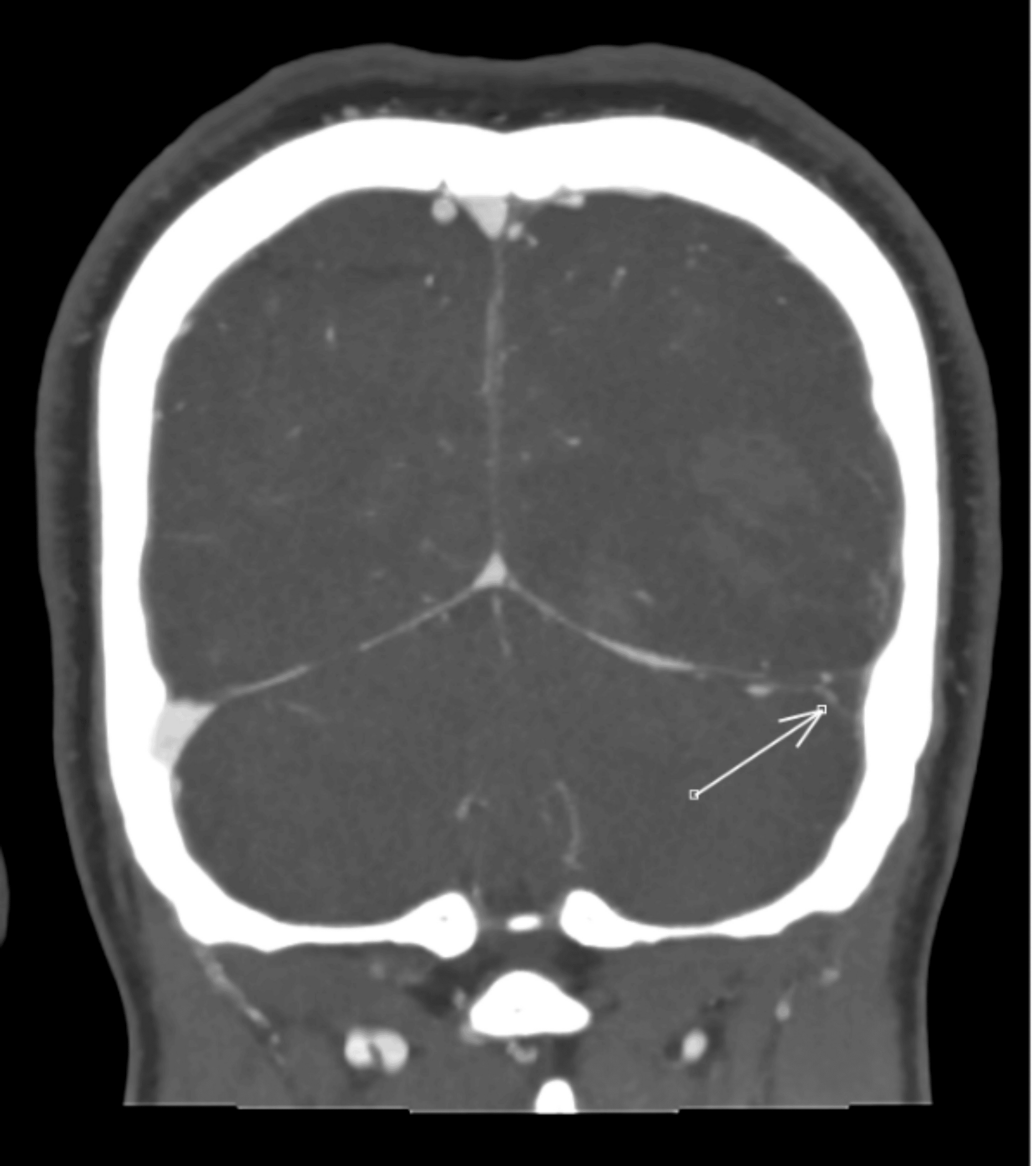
Coronal CT venogram demonstrating cerebral venous sinus thrombosis CT venogram demonstrating a filling defect within the left transverse sinus in keeping with cerebral venous sinus thrombosis. CT: computed tomography
